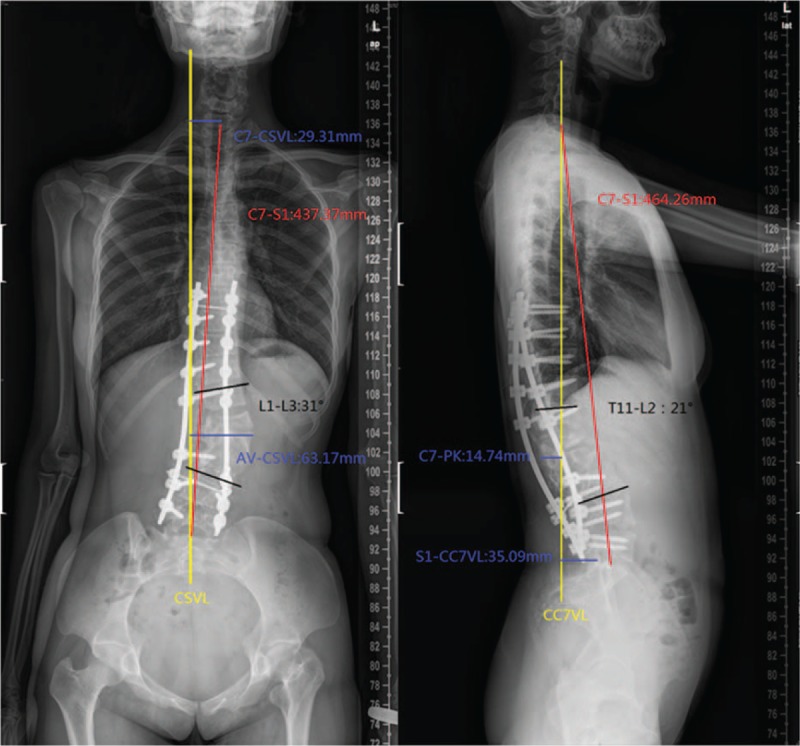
Lateral and anteroposterior X-ray, 3-month postoperative follow-up.
